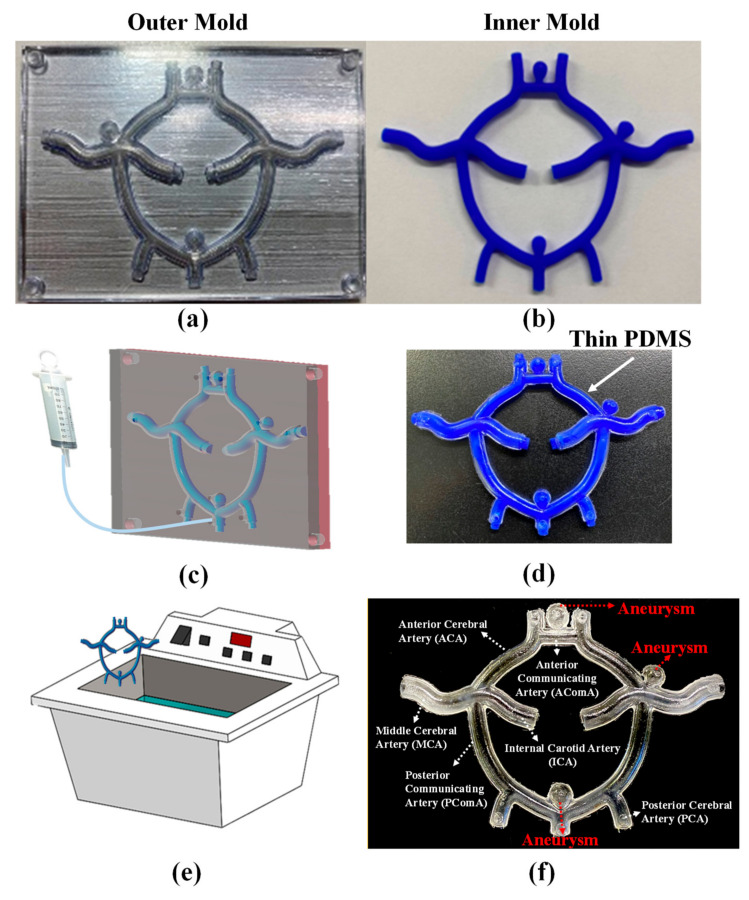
(a) The two outer (clamshell) molds fabricated using a commercial 3D printer (Objet30 Dental Prime, Stratasys) with a biocompatible material (MED 610, Stratasys); (b) the inner wax mold fabricated using a commercial 3D printer (Projet 3500 CPX Max, 3D Systems) with a wax material (Visijet Hi-Cast, 3D Systems); (c) PDMS was slowly injected into the gap between the molds until the gap was completely filled; (d) the PDMS casting (with inner mold still intact) following removal of the outer molds; (e) dissolution of the inner wax mold was facilitated using ultrasonication, as reported previously [23]; (f) the resulting Circle of Willis with major corresponding arteries.
